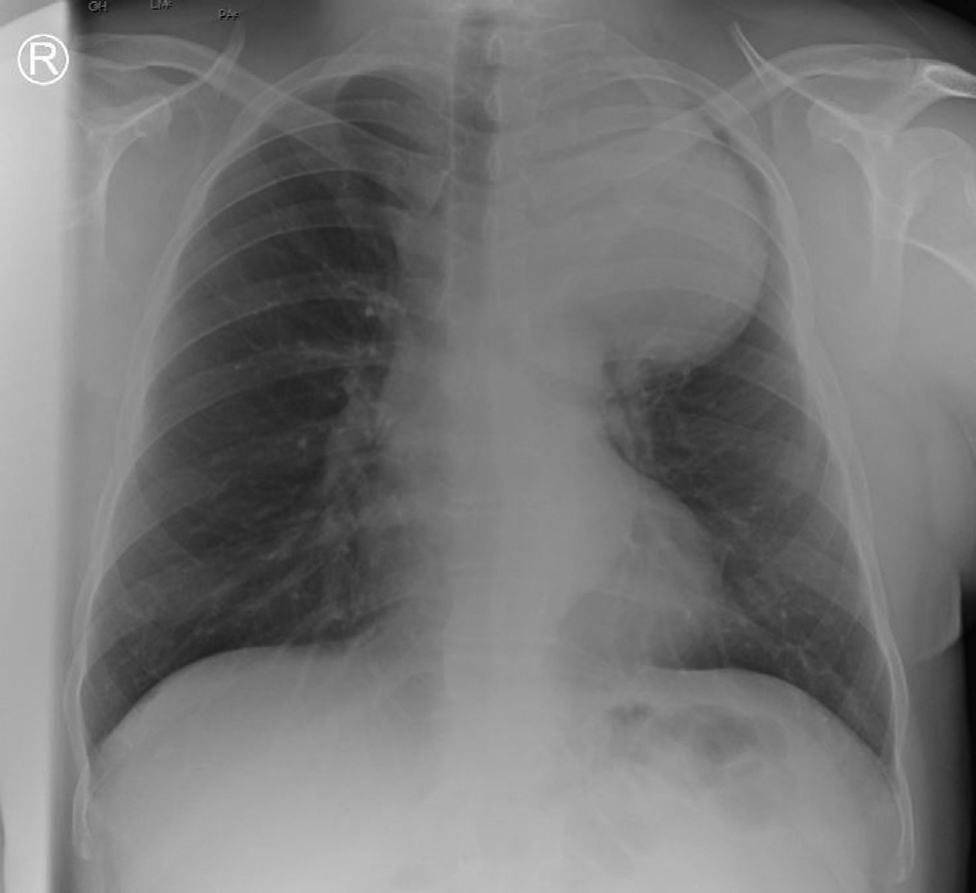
Chest X-ray showed evidence of a widened upper mediastinum with smooth contours.
